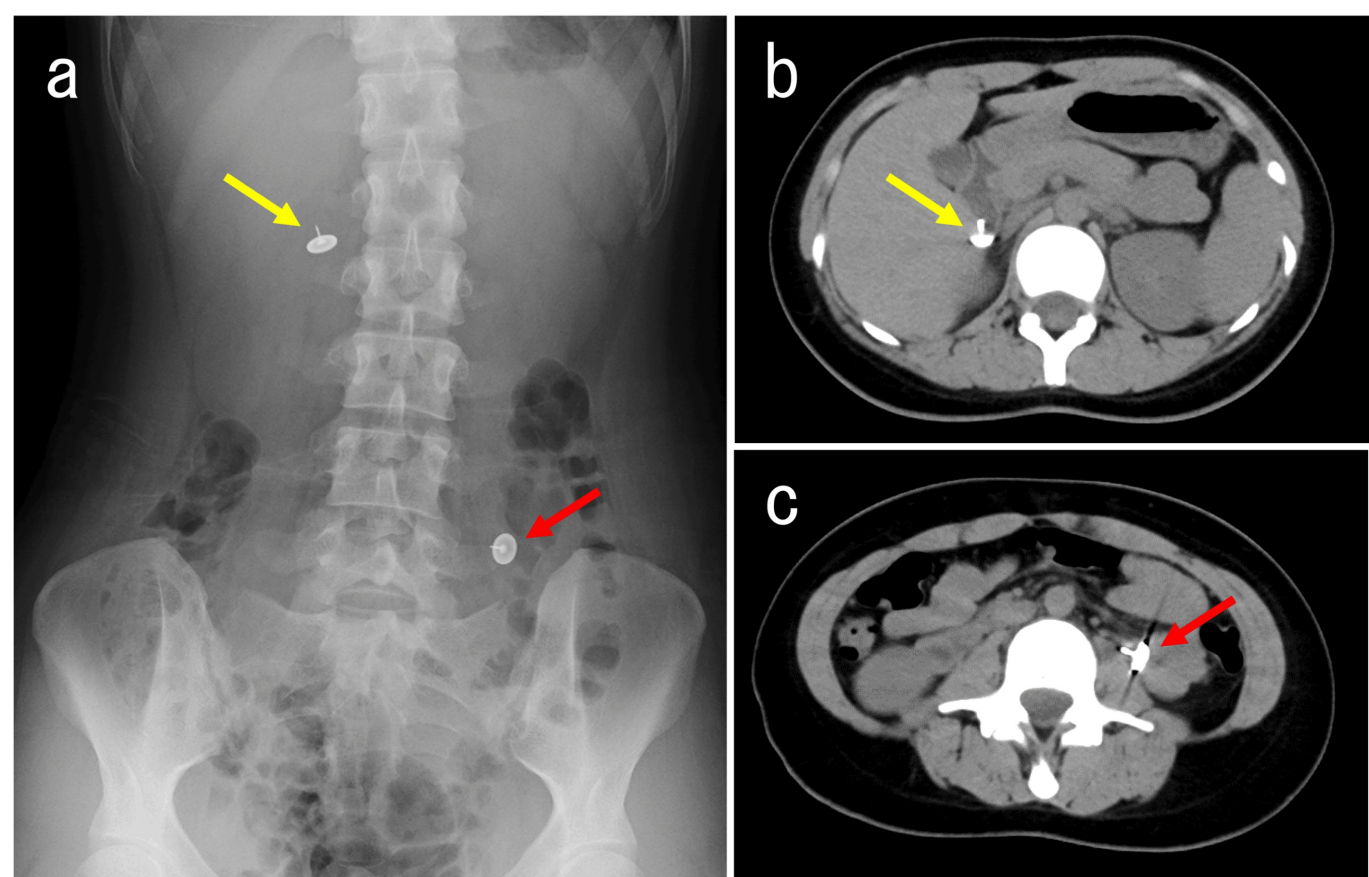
An abdominal X-ray and computed tomography imaging (a) An initial abdominal X-ray revealed two thumbtacks: one in the right upper abdomen (yellow arrow) and the other in the left lower abdomen (red arrow). (b, c) CT scans were performed to accurately locate the foreign bodies and rule out complications such as perforation. The scans identified one thumbtack in the descending duodenum (yellow arrow) and the other in the proximal jejunum (red arrow). No evidence of free air suggestive of gastrointestinal perforation was detected. Endoscopic retrieval was deemed infeasible, and conservative management was chosen.
